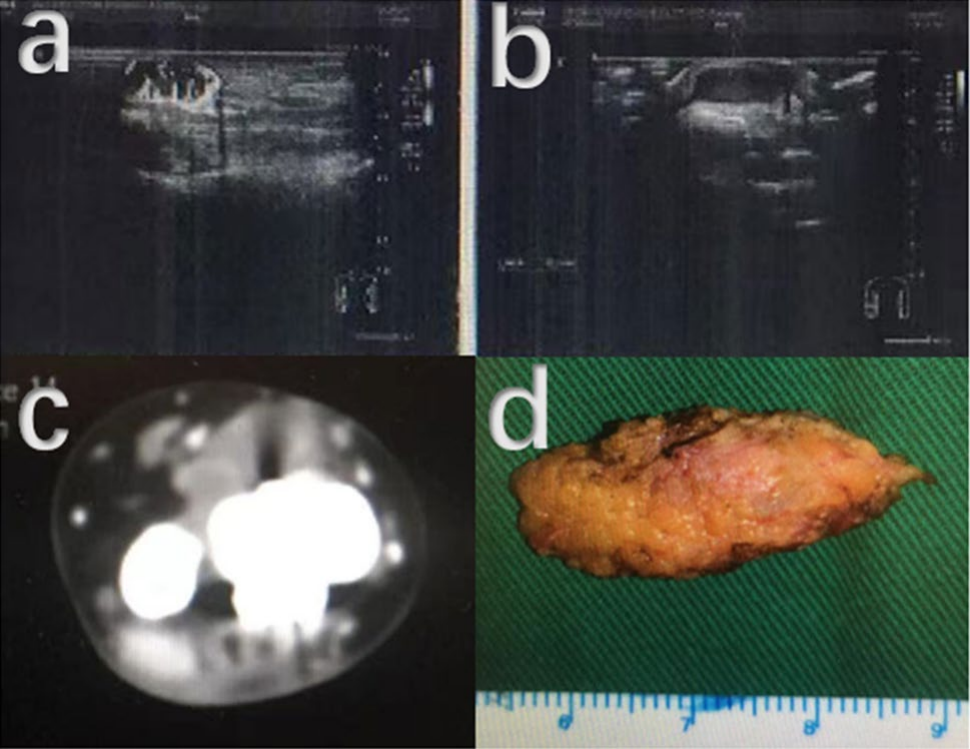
Re-recurrence of soft tissue occurred in the 22 years old female patient with GCTB located in distal radius. a and b A hypoechoic mass was detected 5 months after excision of the first recurrence. c CT examination before operation for the twice recurrence of soft tissue. d The resected soft tissue lesion
